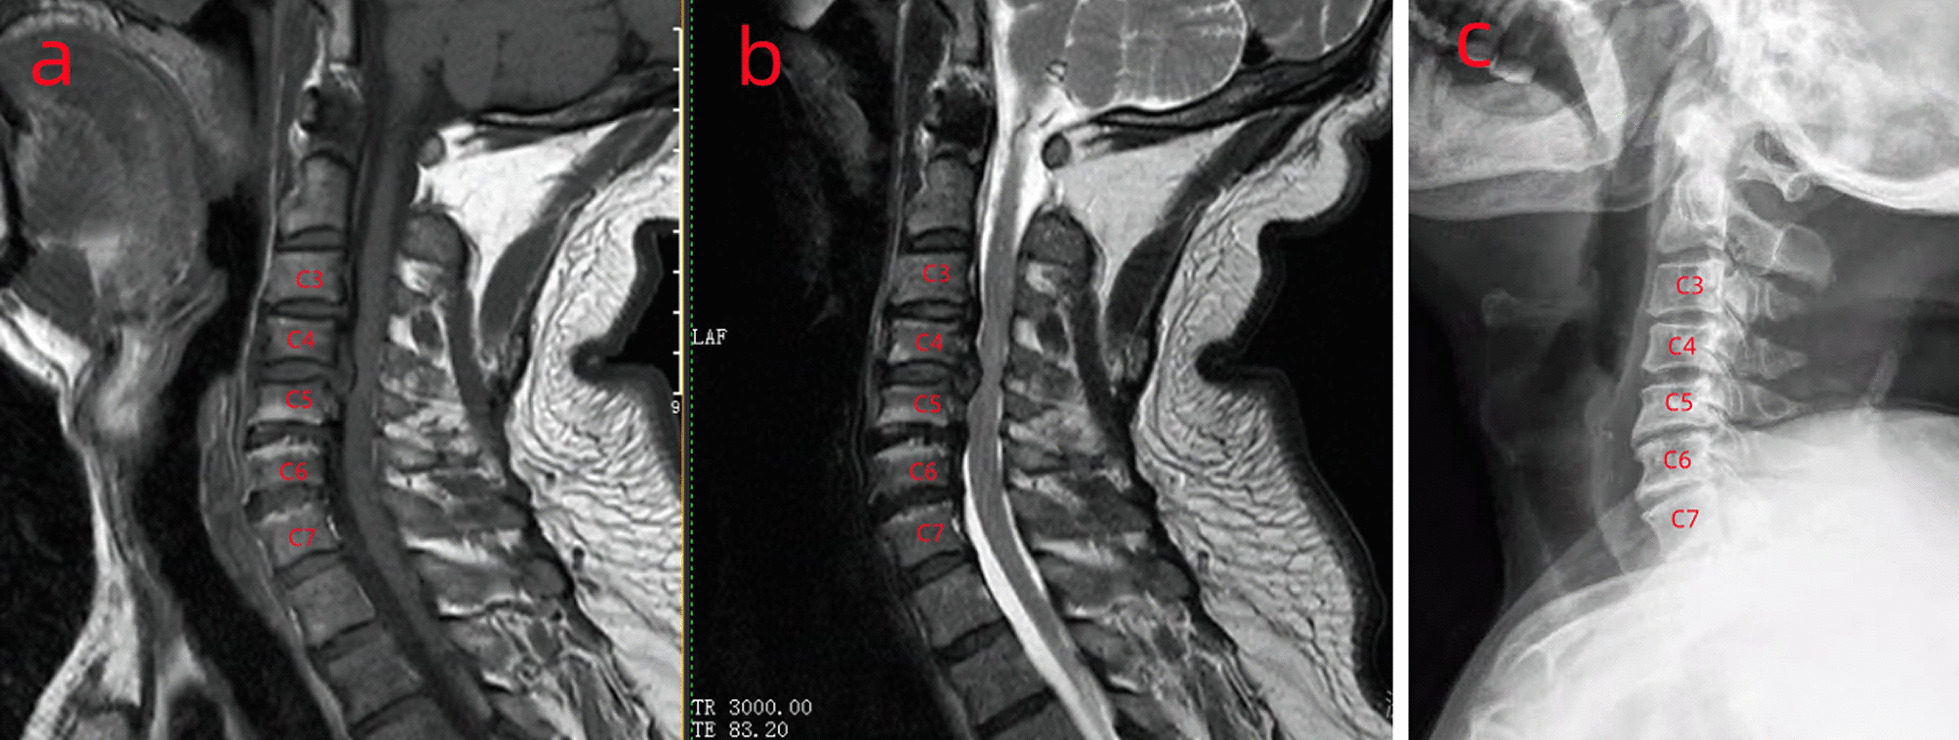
Patient; 60-year-old man, T1-weighted (a) and T2-weighted (b) magnetic resonance images of the cervical end-plates showing the presence of type II Modic changes (indicated by high signal on T1 and T2) on C5–6 level. C4–5 was defined as the adjacent cephalad level, and C3–4 with grade 2 was defined as the control. X-ray showed anterior osteophyte formation on C5–6 level (c)
